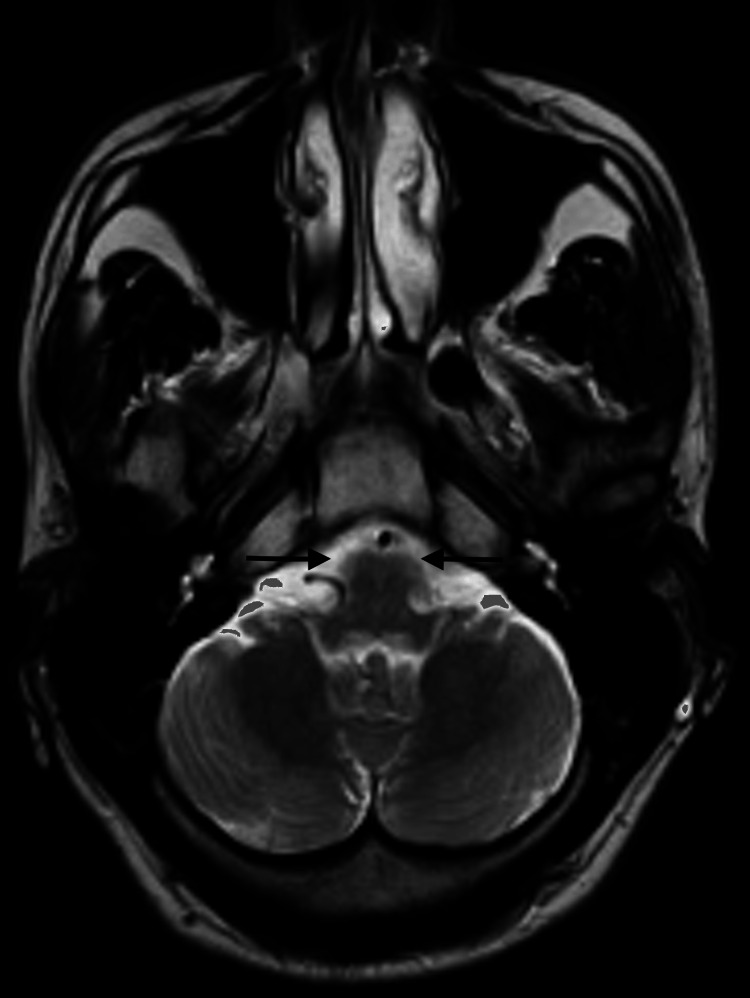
Contrast-enhanced MRI: An initial study. Axial T2 bulb. Bilateral and symmetrical hyperintensity of the mesencephalic tectum and, to a lesser extent, the bulbar tectum is observed on T2-weighted images (arrows).
